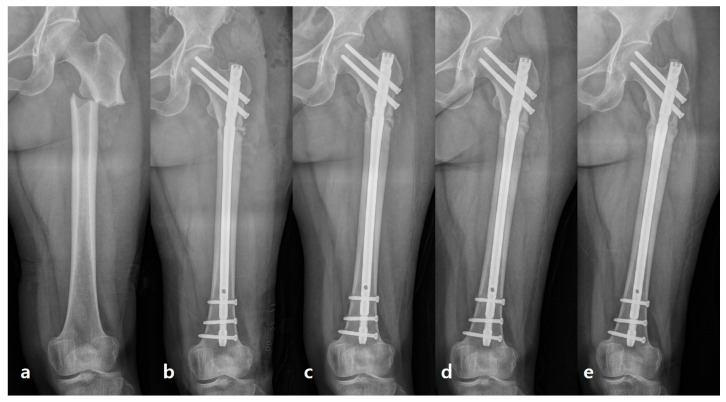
Serial anteroposterior radiographs of a 60-year-old woman with a subtrochanteric complete atypical femoral fracture fixed with femoral recon nail. (a) preoperative, (b) immediate postoperative, (c) 6 weeks follow-up, (d) 14 weeks follow-up, (e) 22 weeks follow-up X-ray shows progressive callus formation and consolidation of decorticated bone chips.
